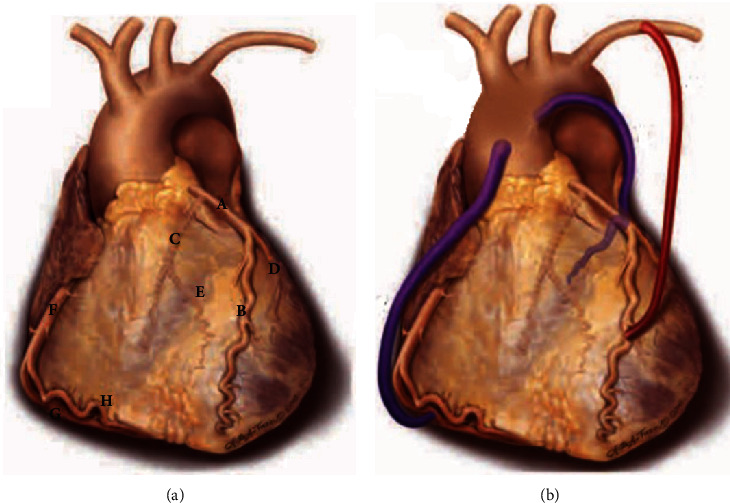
(a) Normal anatomy of the coronary arteries: (A) left coronary artery, (B) left anterior descending artery, (C) left circumflex artery, (D) diagonal artery, (E) obtuse marginal branch of the left circumflex artery, (F) right coronary artery, (G) posterior descending artery, and (H) acute marginal branch of the right coronary artery. (b) Left internal mammary artery graft from its origin at the subclavian artery proximally and grafted to the left anterior descending artery distally (in red) [4]. This figure is reproduced from Frazier, A., Qureshi, F., Read, K. M., Gilkeson, R. C., Poston, R. S., & White, C. S. (2005). Coronary Artery Bypass Grafts: Assessment with Multidetector CT in the Early and Late Postoperative Settings. Radiographics, 25(4), 881-896 (under the Creative Commons Attribution License/public domain).
